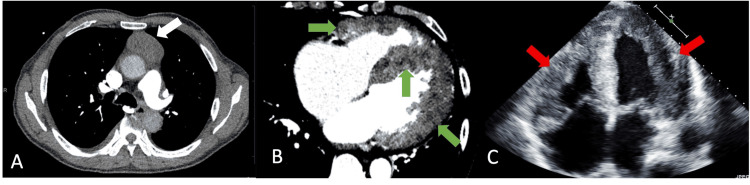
Admission CT scans and TTE (A) Axial pulmonary artery CTA demonstrates an anterior mediastinal mass of soft tissue attenuation (white arrow) measuring 5.2 x 2.8 x 5.2 cm (AP x TV x CC) at the expected location of the thymus (smaller mass not pictured). (B) Axial CT of the abdomen and pelvis with contrast performed on the same day, zoomed in on the heart, demonstrates diffuse myocardial thickening of the right and left ventricles with heterogeneous enhancement (green arrows). (C) Apical four-chamber view TTE demonstrates moderate to severe right ventricular hypertrophy and severe left ventricular hypertrophy (red arrows). Left ventricular ejection fraction was approximately 20%. Left ventricular myocardium was reported to have a speckled appearance, suggesting a diffuse infiltrative process. CT: computed tomography, TTE: transthoracic echocardiography, CTA: computed tomography angiography, AP: anteroposterior, TV: transverse, CC: craniocaudal.
